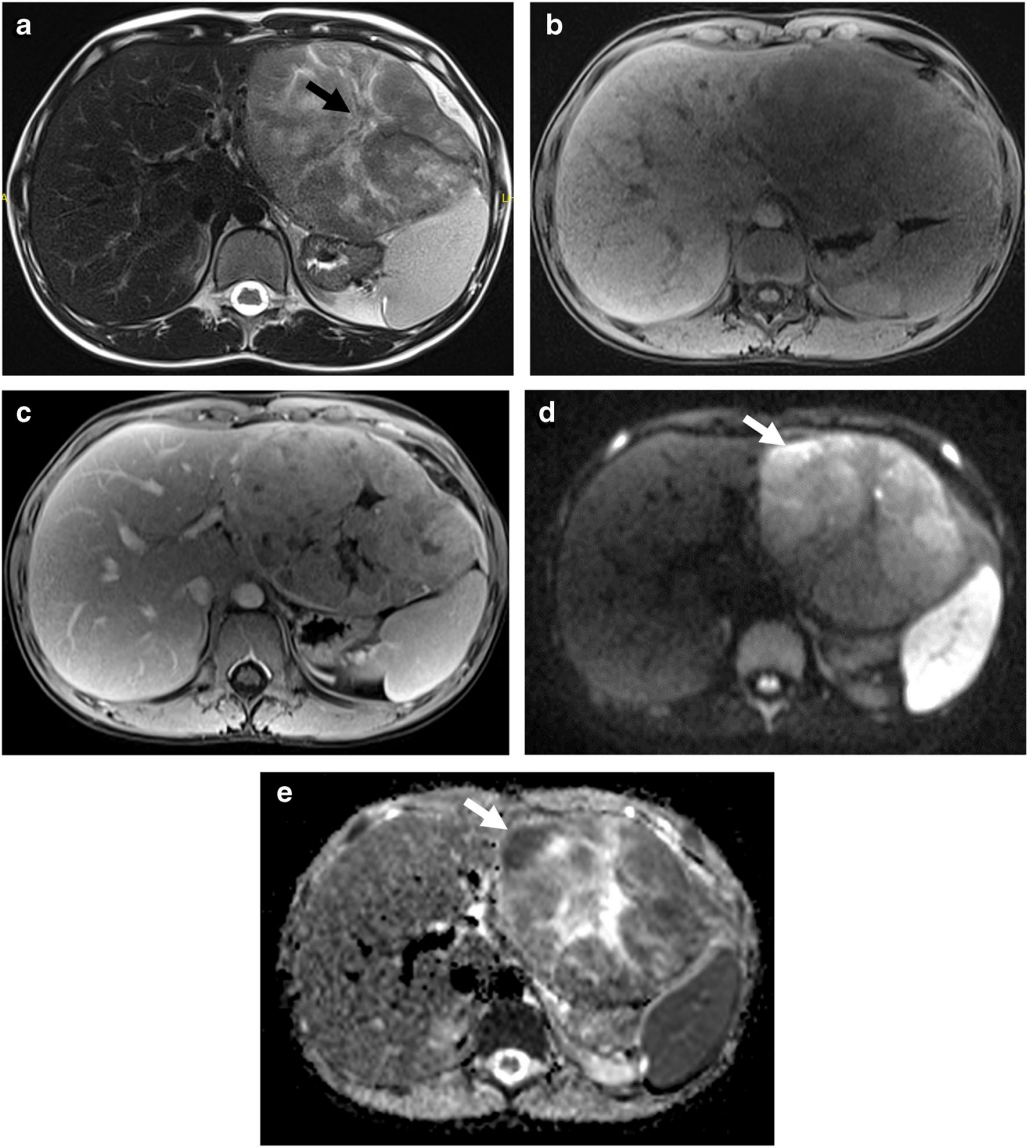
Fibrolamellar hepatocellular carcinoma in a 12-year-old boy. a–c Axial T2-weighted (a), and precontrast (b) and postcontrast (c) T1-weighted images show large heterogeneous mass (*arrow* in a) in the left liver lobe with central scar. d, e Axial diffusion weighted image at *b*=800 s/mm^2^ (a) and ADC map (b) demonstrate restricted diffusion (*arrows*)
